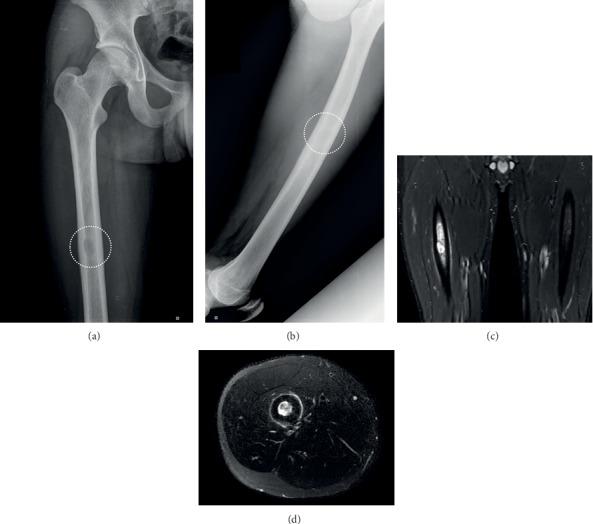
The initial plain radiograph of the femur ((a) anteroposterior and (b) lateral) depicting (dotted line) a centrally located lytic lesion at the femoral diaphysis with concomitant reactive cortical thickness. The initial MRI scan of the lesion. (c) Short T1 reverse- (STIR-) weighted image (WI) coronal view. (d) T2WI axial view (see text for more details).
